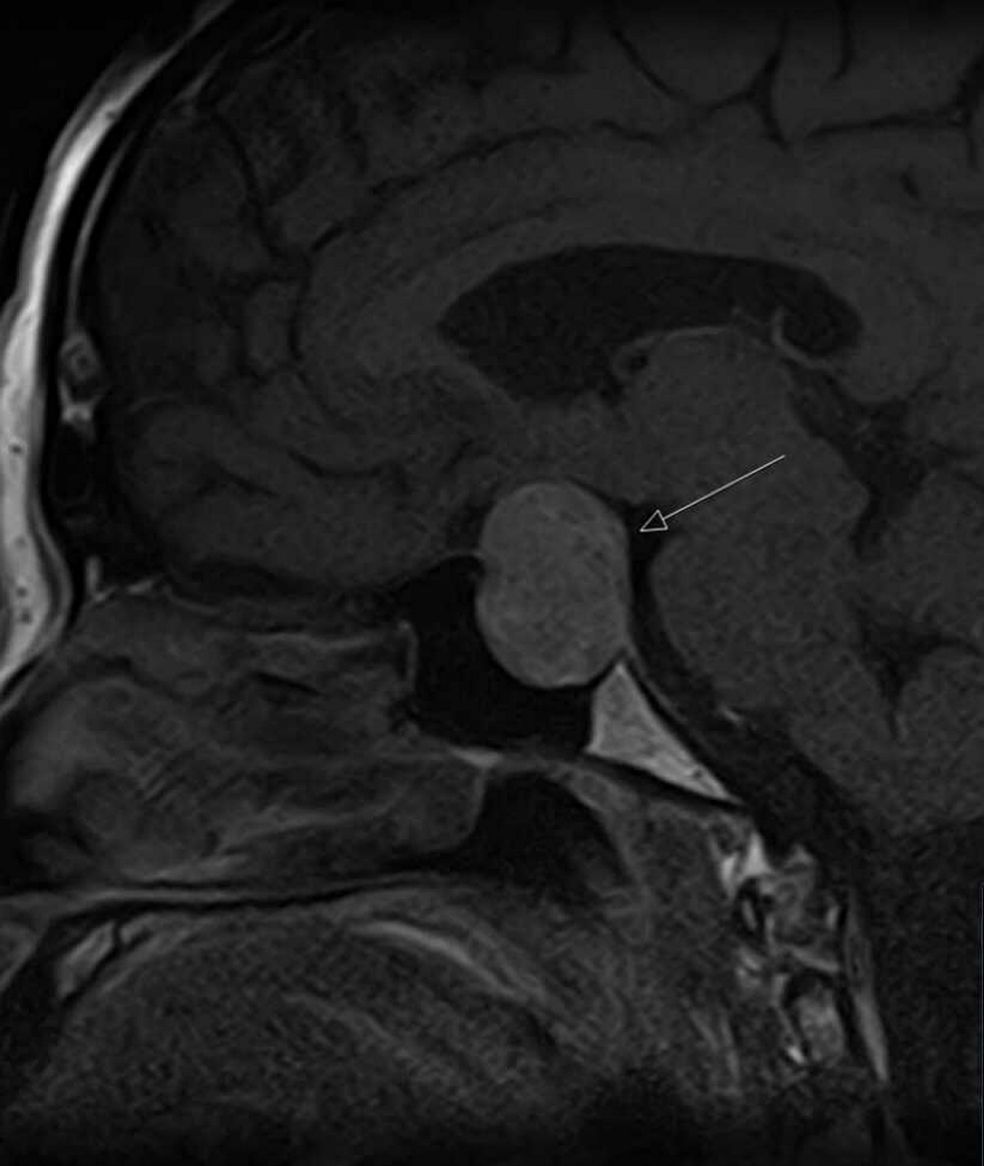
MRI showing pituitary tumor
